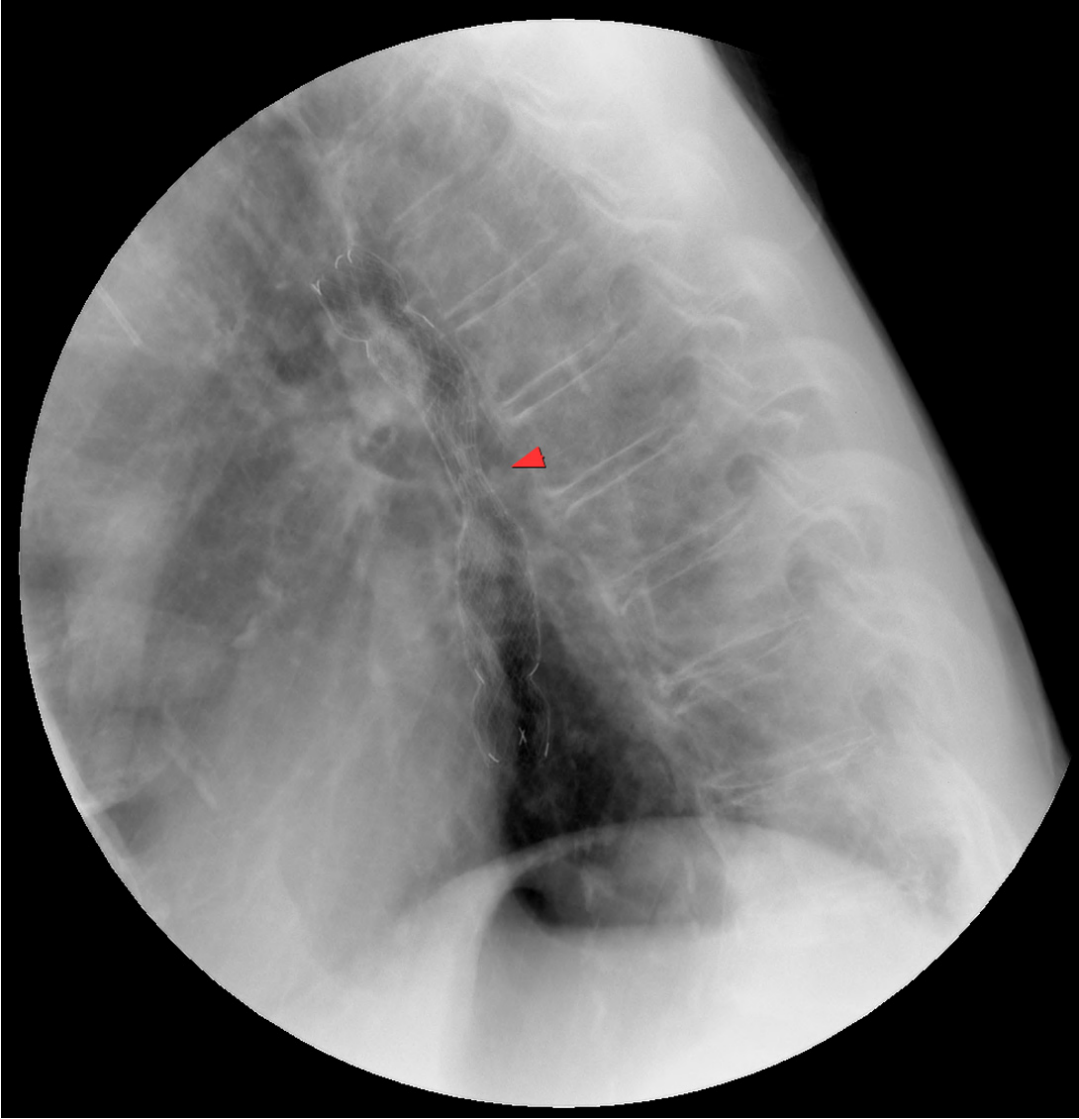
Lateral chest radiograph after self‐expandable metallic stent insertion (red arrow head pointing to the area of stenosis).
